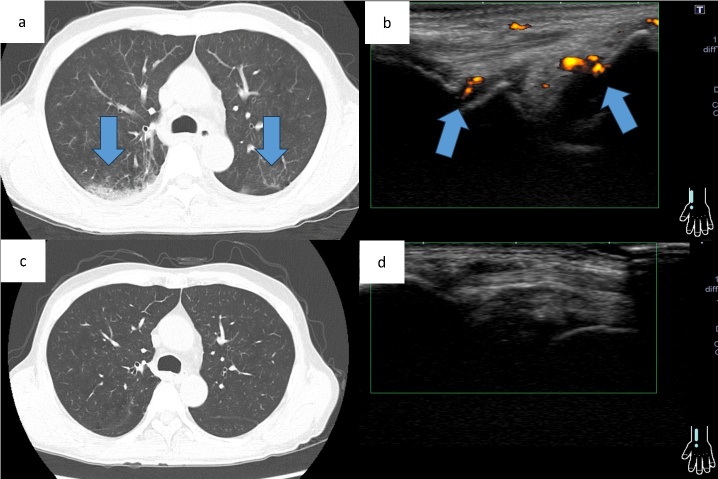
Chest computed tomography and joint ultrasonography images before and after transplantation. Chest computed tomography image before transplantation showing numerous frosted shadows in the periphery of both lungs, (b) Joint ultrasonography image before transplantation showing increased blood flow in the synovial area and arthritis, (c) Chest computed tomography image after transplantation showing the disappearance of the numerous frosted shadows in the periphery of both lungs, (d) Joint ultrasonography image after transplantation showing the disappearance of the arthritis
